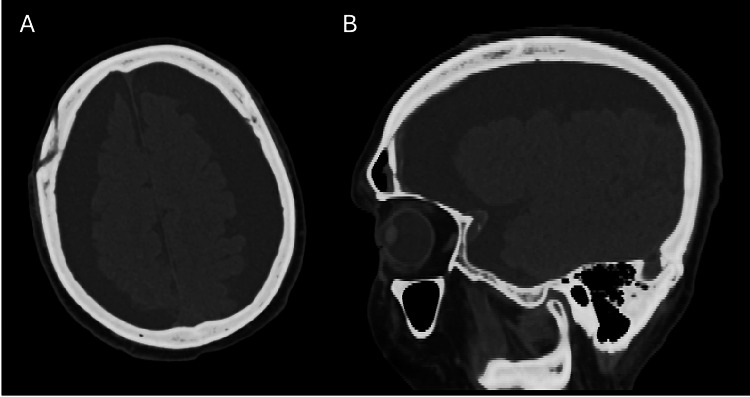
(A) Axial and (B) sagittal preoperative CT imaging displaying the bilateral subdural hygroma
